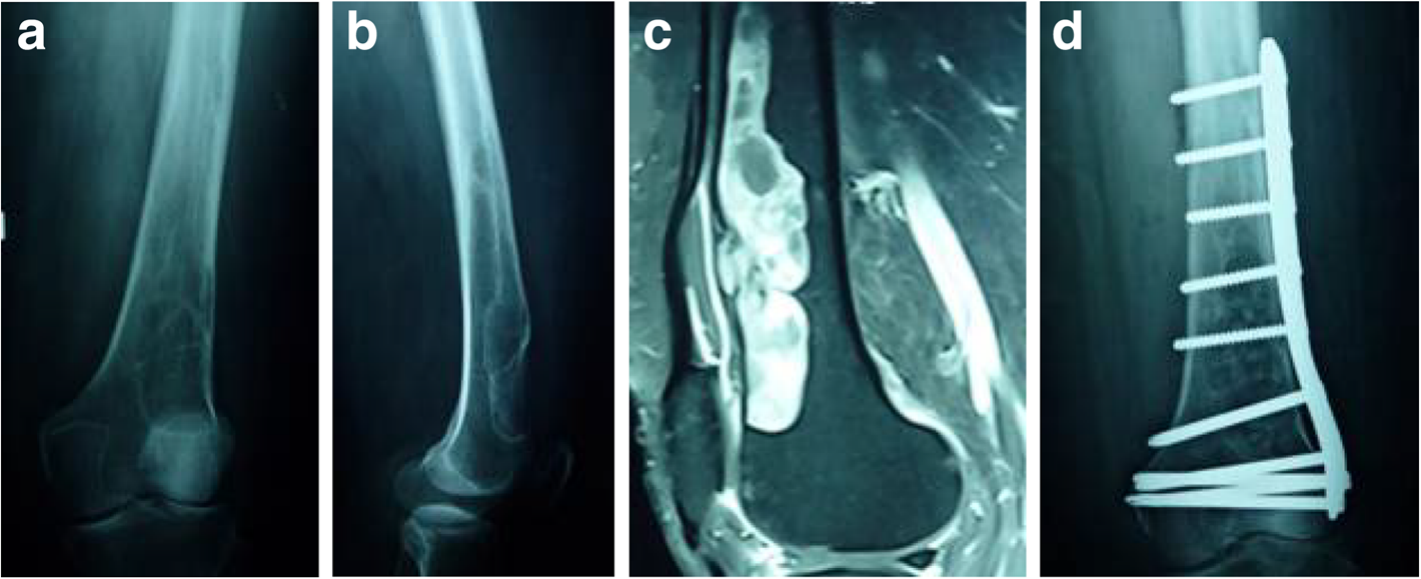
a Anteroposterior and b lateral radiographs of the distal femur showing the osteolytic lesion in the femoral cortex. c T1-weighted magnetic resonance imaging shows lobulated tumor. d Radiograph shows prophylactic internal fixation with a distal femoral plate after evacuation of tumor
